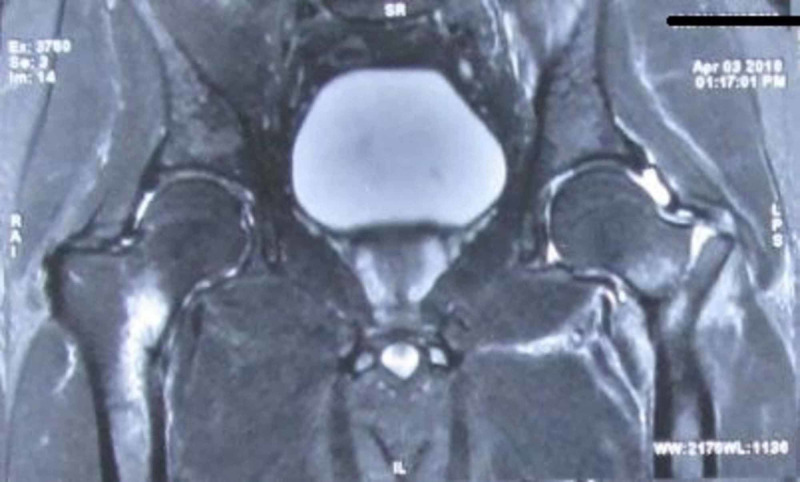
MRI showing displaced basicervical neck of femur fracture on the left side and incomplete neck of femur fracture on the right side. MRI: Magnetic resonance imaging.
